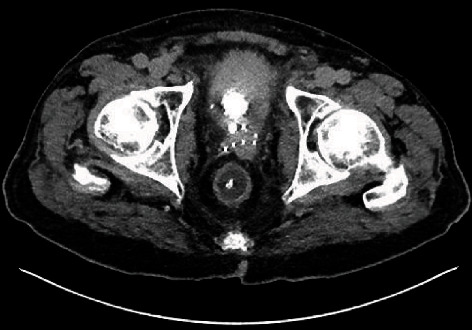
Axial CT demonstrating catheter placement in rectum.
